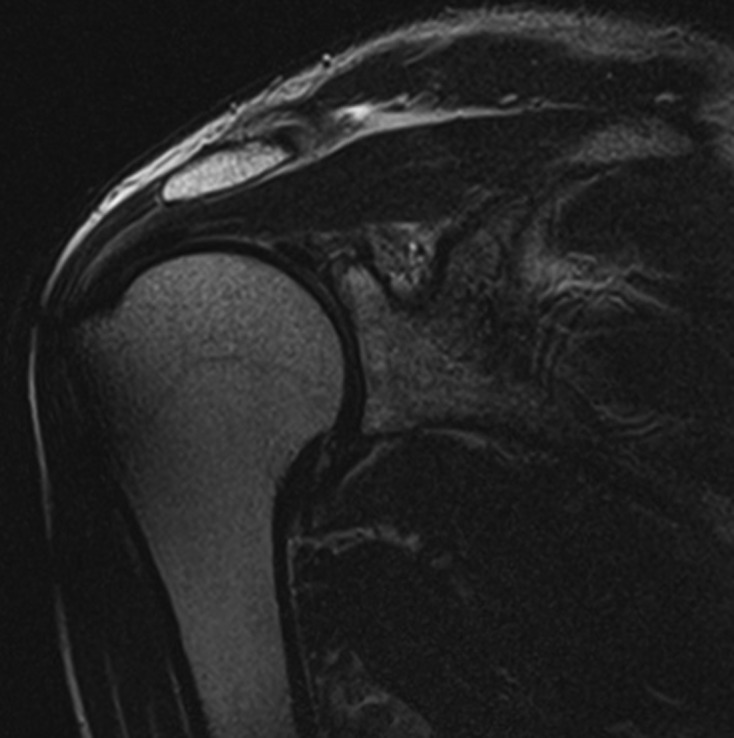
Postoperative T2-weighted MRI scan demonstrating disappearance of the spinoglenoid cyst.
